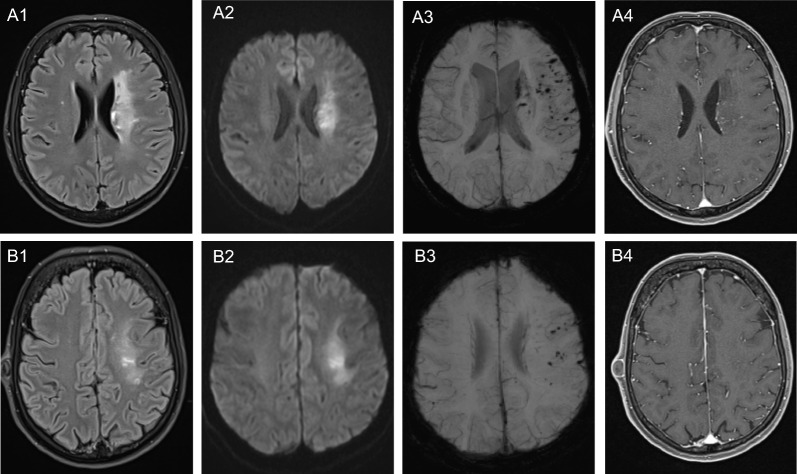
MRI findings in CAA-ri at the level of cella media (A1-A4) and centrum semiovale (B1-B4). FLAIR images (A1-B1) disclose a left-sided periventricular leukoencephalopathy, with correlating diffusion restriction (A2-B2); the DWI signal seems rather indicative of prominent T2-shine through effect than plain stroke. Hemosiderin deposits are depicted (A3-B3), extending from the periventricular region to the left frontoparietal cortex. In addition, subtle perivascular enhancement is noted in the periventricular area (A4-B4)
